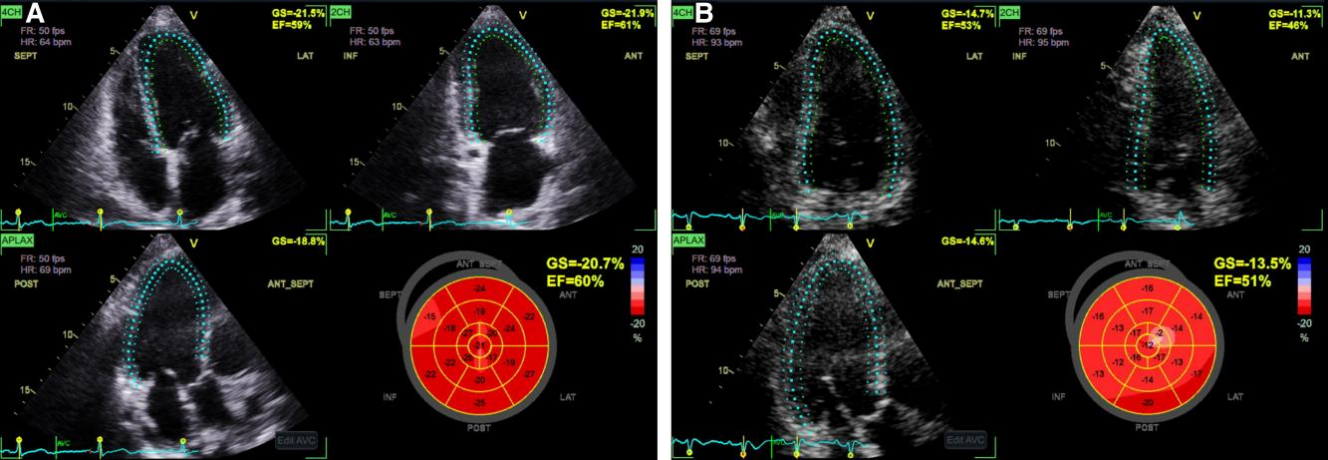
(*A*) Baseline echocardiogram showing an estimated ejection fraction of 60%–65% with a global longitudinal strain of 20.7%. (*B*) Admission echocardiogram with an apparent mild reduction in ejection fraction to 55%–60% (in-line video 2) and a fall in GLS to 13.5%. No regional wall motion abnormalities were noted.
